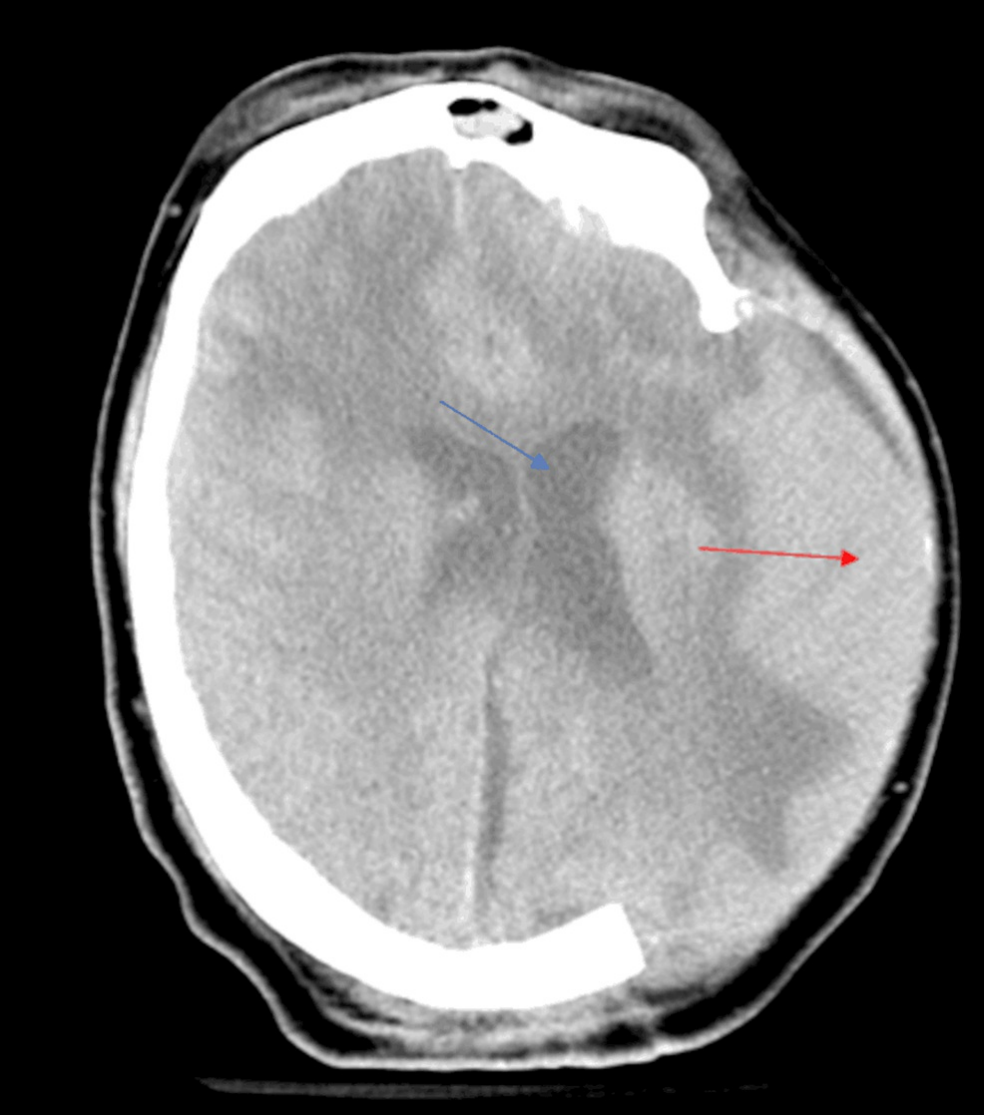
Axial head CT The view shows increased ventricular size (blue arrow) and parenchymal protrusion through the craniectomy defect (red arrow) with diffuse parenchymal edema in bilateral frontal lobes when the lumbar drain was not functioning.
